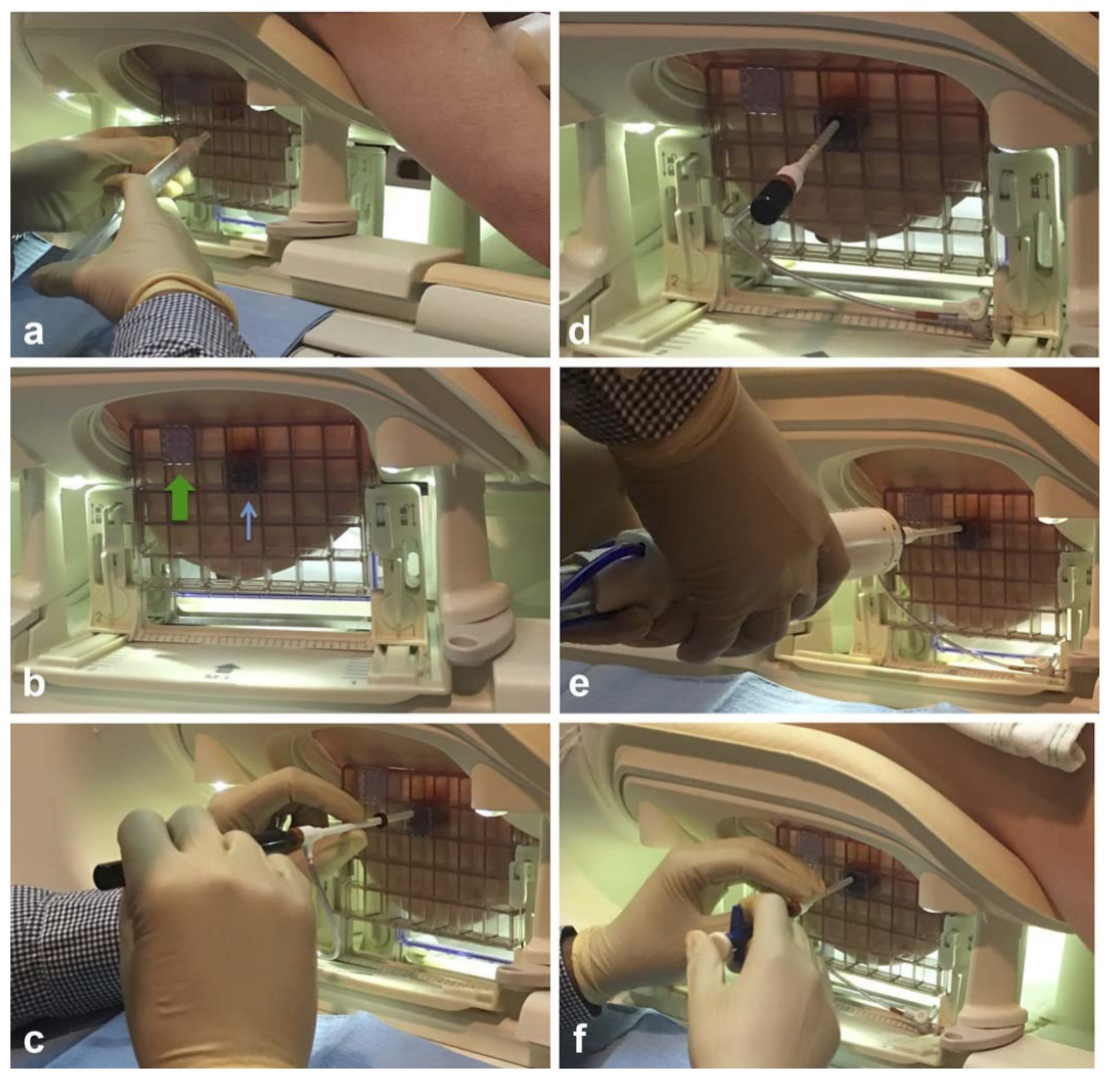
Example of typical steps involved when performing an MRI-guided breast biopsy. After determining the appropriate grid space for the skin entry site, the skin is cleansed and anesthetized (A), and local anesthetic is administered into the deeper breast tissues. The tunneled needle guide is then placed in the grid space where the biopsy (thin blue arrow) noting location relative to the fiducial (wide green arrow) (B). Using a “twist and push” motion, the plastic introducer sheath is placed into the breast through the appropriate hole in the tunneled needle guide with the cutting introducer stylet inside the sheath until the sheath reaches the appropriate depth as indicated by the rubber stopper (C). The cutting introducer stylet is subsequently replaced with a plastic obturator, and the patient is placed back in the bore of the MRI scanner so that a confirmatory T1-weighted sequence can be performed to confirm the appropriate location (D). The table is then brought out of the bore and the plastic obturator is replaced with the vacuum-assisted breast biopsy (VABB) device and multiple cores are obtained (E). After the sampling is complete, the VABB device is removed and a biopsy marker clip is deployed through the sheath (F). Modified and reprinted from McGrath AL, Price ER, Eby PR, Rahbar H. MRI-guided breast interventions. J Magn Reson Imaging. 2017. 46(3): 631-645. Copyright © 2017 International Society for Magnetic Resonance in Medicine.
